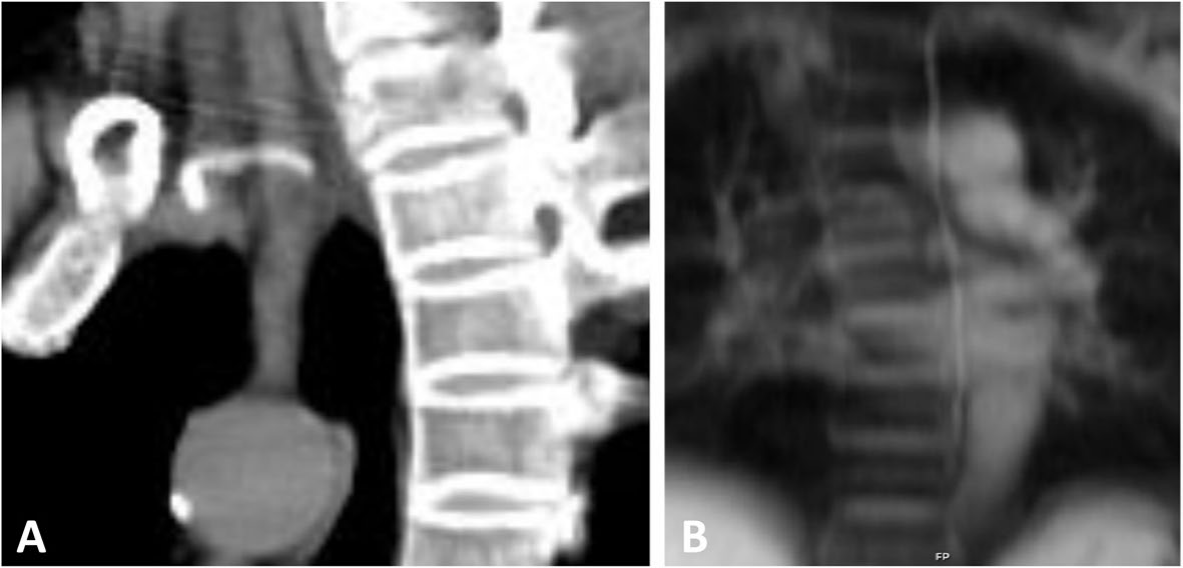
Follow up images. A CT scans 6 months post-stenting revealed the stent was in the right position. B DCMRL after one year showed the patency of thoracic duct
